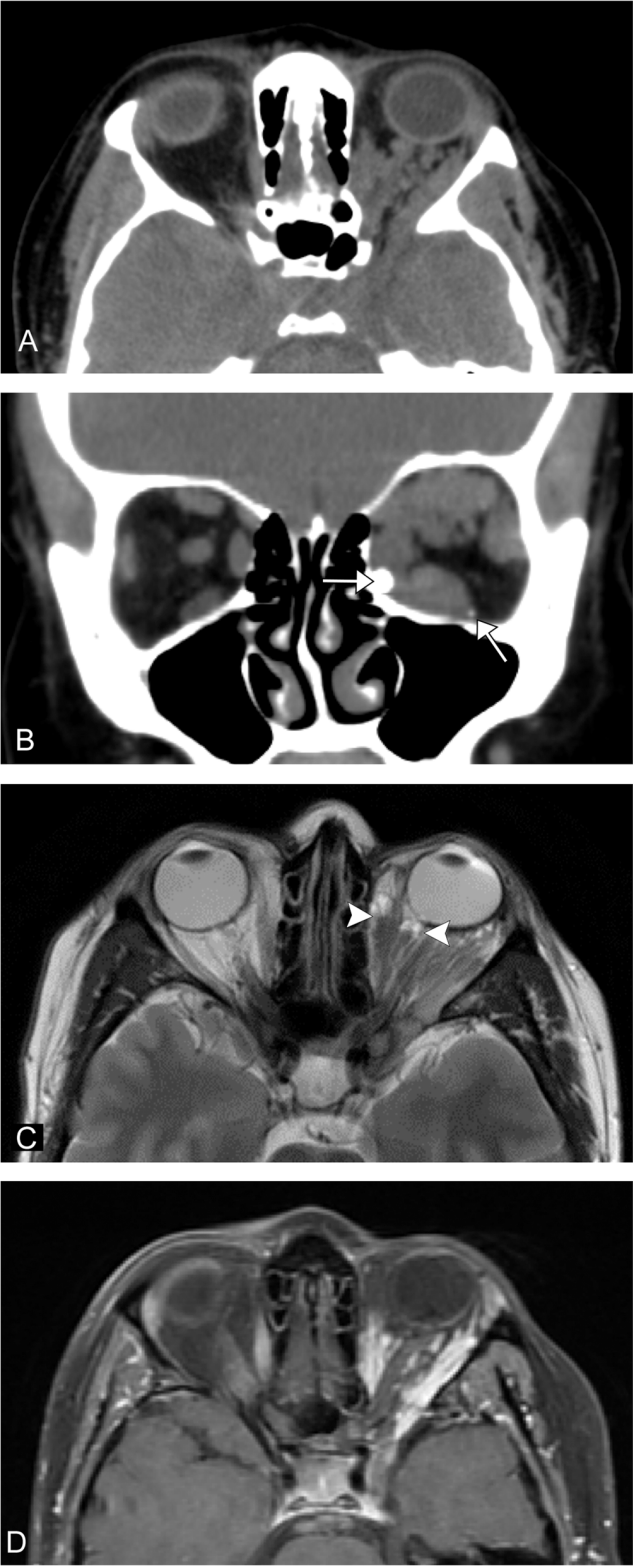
Venolymphatic malformation. A 31-year-old female presents with left eye fullness. a Axial CT image demonstrates a serpiginous lesion within the left orbit causing proptosis. b Coronal CT image demonstrates a few phleboliths at the inferomedial margin (arrows). c Axial T2-weighted image shows fluid-fluid levels within the lesion (arrowheads). d Axial T1 post-contrast fat-saturated image shows enhancement of portions of the lesion
